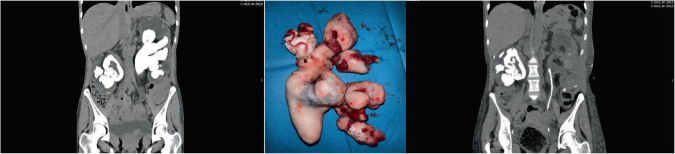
Removal of a massive staghorn calculi by left anatrophic nephrolithotomy and pyelolithotomy. A: Pre-operative CT scan showing bilateral staghorn calculi and dimensional asymmetry between the kidneys. The right kidney appeared with a lower volume and higher parenchymal rarefaction. B: Removed staghorn calculi. C: CT scan showing complete removal of the calculi from the left kidney.
